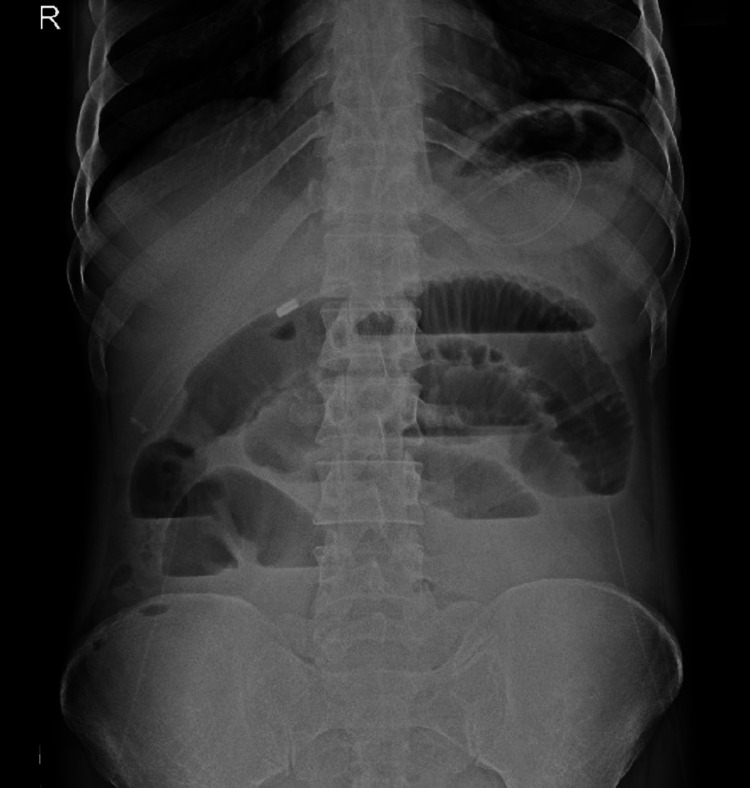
Radiograph of the abdomen in standing view The erect abdominal X-ray revealed dilated small bowel loops with multiple air-fluid levels, suggestive of small bowel obstruction.
